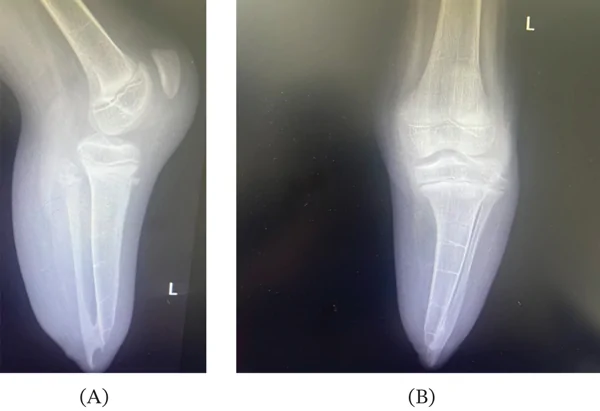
(A) Anteroposterior and (B) lateral radiographs of the left residual limb demonstrating below‐knee amputation with distal tibial and fibular osseous overgrowth.
